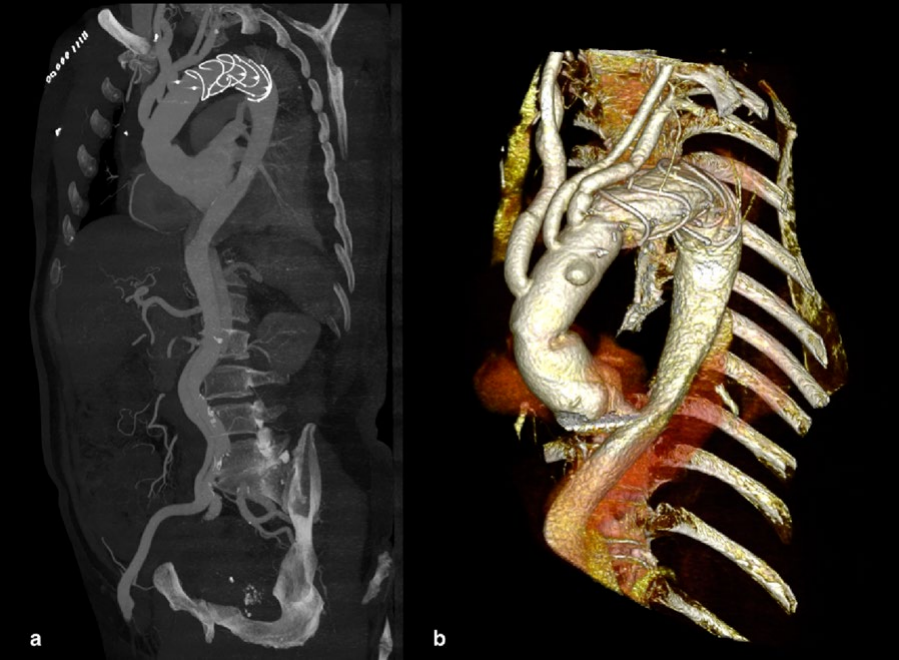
Postoperative CT. Maximum intensity projection (a) and three-dimensional CT (b) showing replacement of ascending aorta, aortic arch and FET in the descending aorta
